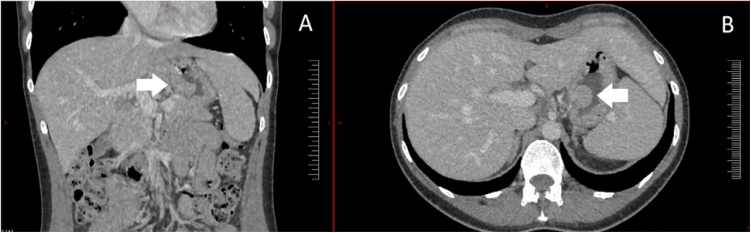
Coronal (A) and axial (B) CT-scan images showing a 25 mm long axis lesion located on the small curvature of the stomach under the cardia.
